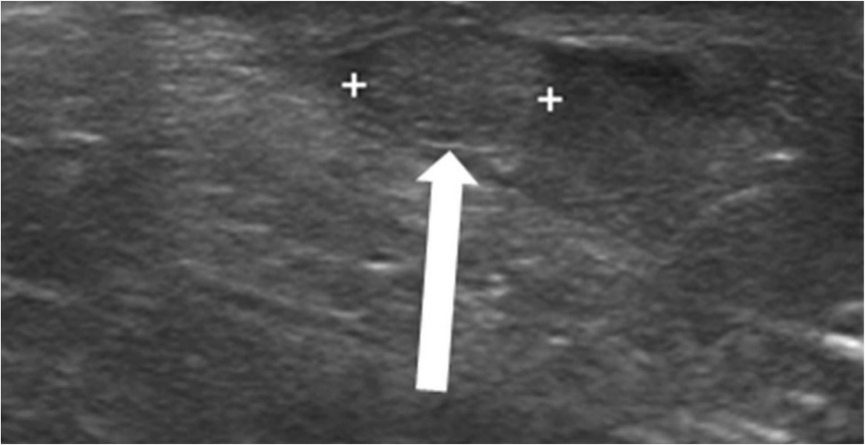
Ultrasound of the right inguinal canal in patient 2. Image shows a 0.7cm oval mass that resembles a testis in its echotexture (white arrow).
